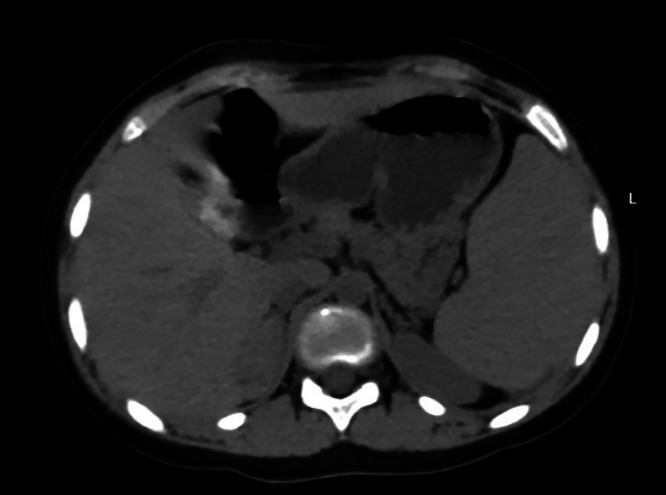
precontrast CT scan of the right adrenal soft tissue lesion (48HU), no fat or calcific content.
